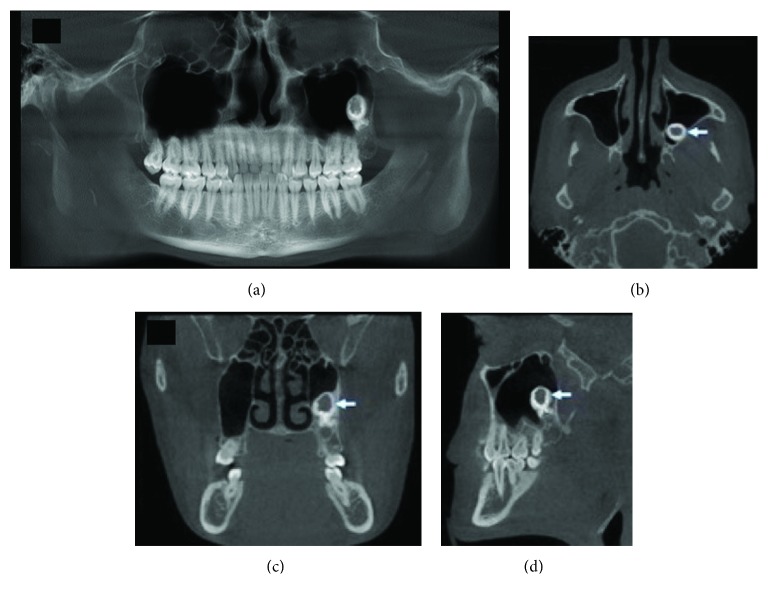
Computed tomography preoperative showing mass associated with the roots of the third molar inside the maxillary sinus. (a) Panoramic view. (b) Axial plane. (c) Coronal plane. (d) Sagittal plane.
